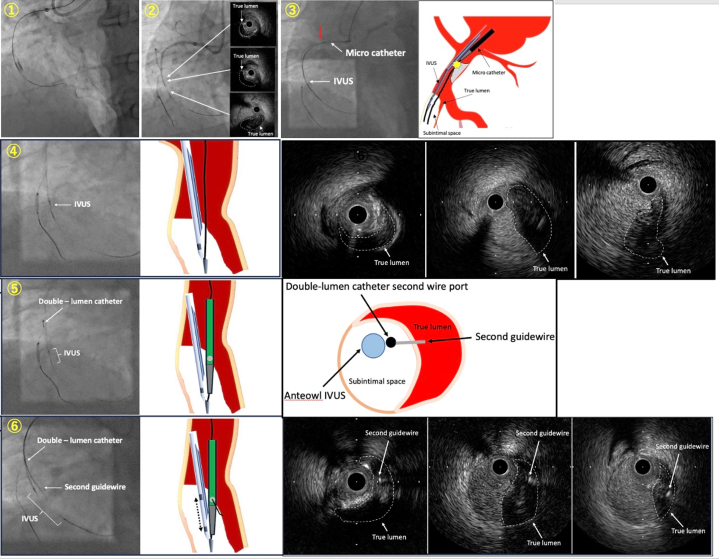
Novel Slipstream and Tip Detection Technique for Efficient and Precise ADR IVUS = intravascular ultrasound.
